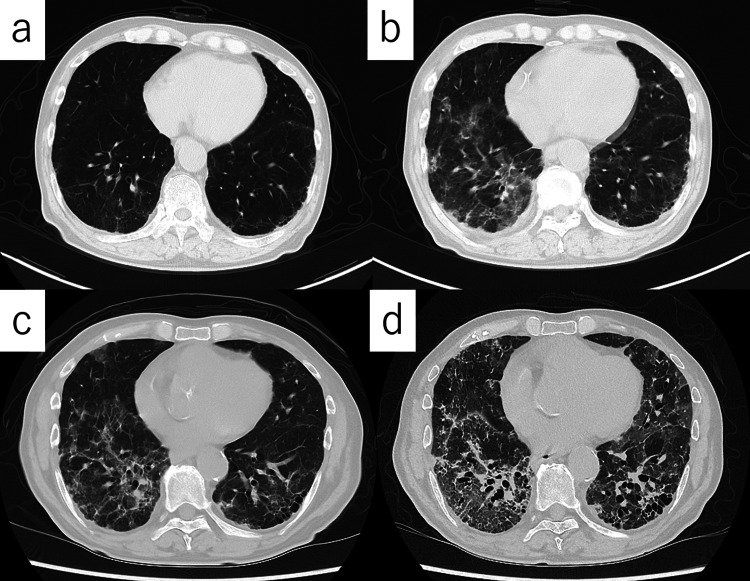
Radiologic course of drug-induced interstitial pneumonitis. Sequential chest CT scans showing the rapid progression of diffuse, non-segmental ground-glass opacities despite corticosteroid therapy (a) Baseline CT prior to symptom onset. (b) Day 0 (on hospital admission): newly developed bilateral ground-glass opacities. (c) Day 11 (after the first course of corticosteroid pulse therapy): no radiologic improvement observed. (d) Day 24 (after the second pulse therapy): bilateral consolidation and traction bronchiectasis further progressed, consistent with fatal drug-induced ILD. CT: computed tomography, ILD: interstitial lung disease
